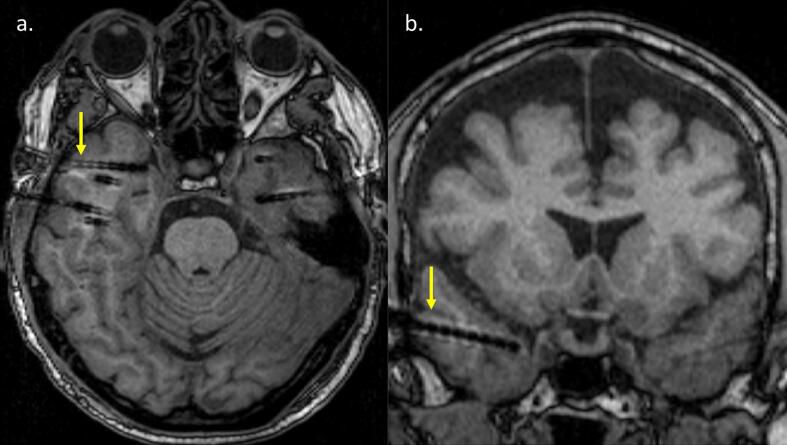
Axial (a) and coronal (b) T1-weighted MPRAGE images showing typical placement of a depth electrode in the right temporal pole (arrows) in a representative patient.
